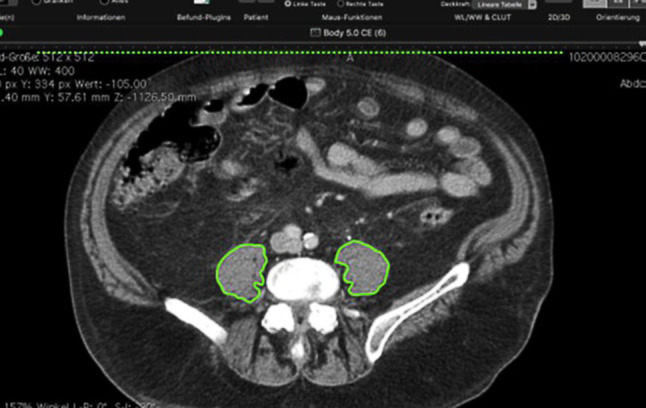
Cross-sectional X-ray image at the L3 level showing measurement of TPA in the psoas muscles (marked *green*)
